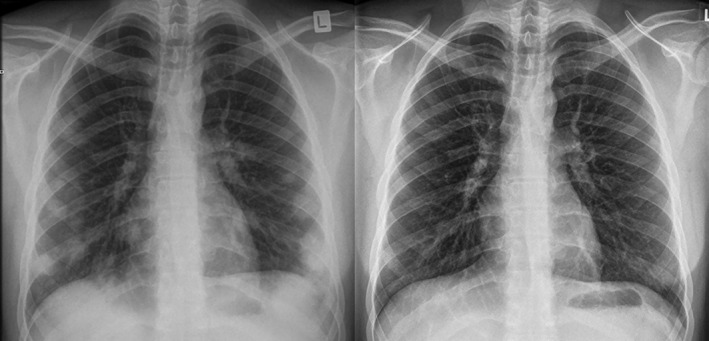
Left – chest radiograph at 2 weeks. Right – chest radiograph at 12 weeks demonstrated resolution.
